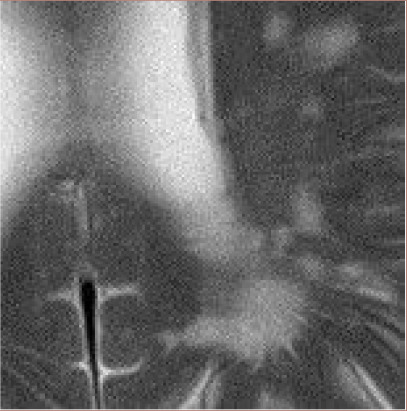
Lacune	Round or ovoid fluid filled cavity (3-15 mm diameter in the axial plane) mostly in subcortical regions; hyperintensity on T2-weighted MRI; decreased signal in FLAIR (often with hyperintense halo) and T1-weighted images. Signal characteristics similar to CSF.	Usually due to infarction in the territory of a single perforating artery, but can also result from small hemorrhages or occlusion of a larger artery (e.g., striatocapsular infarct).
